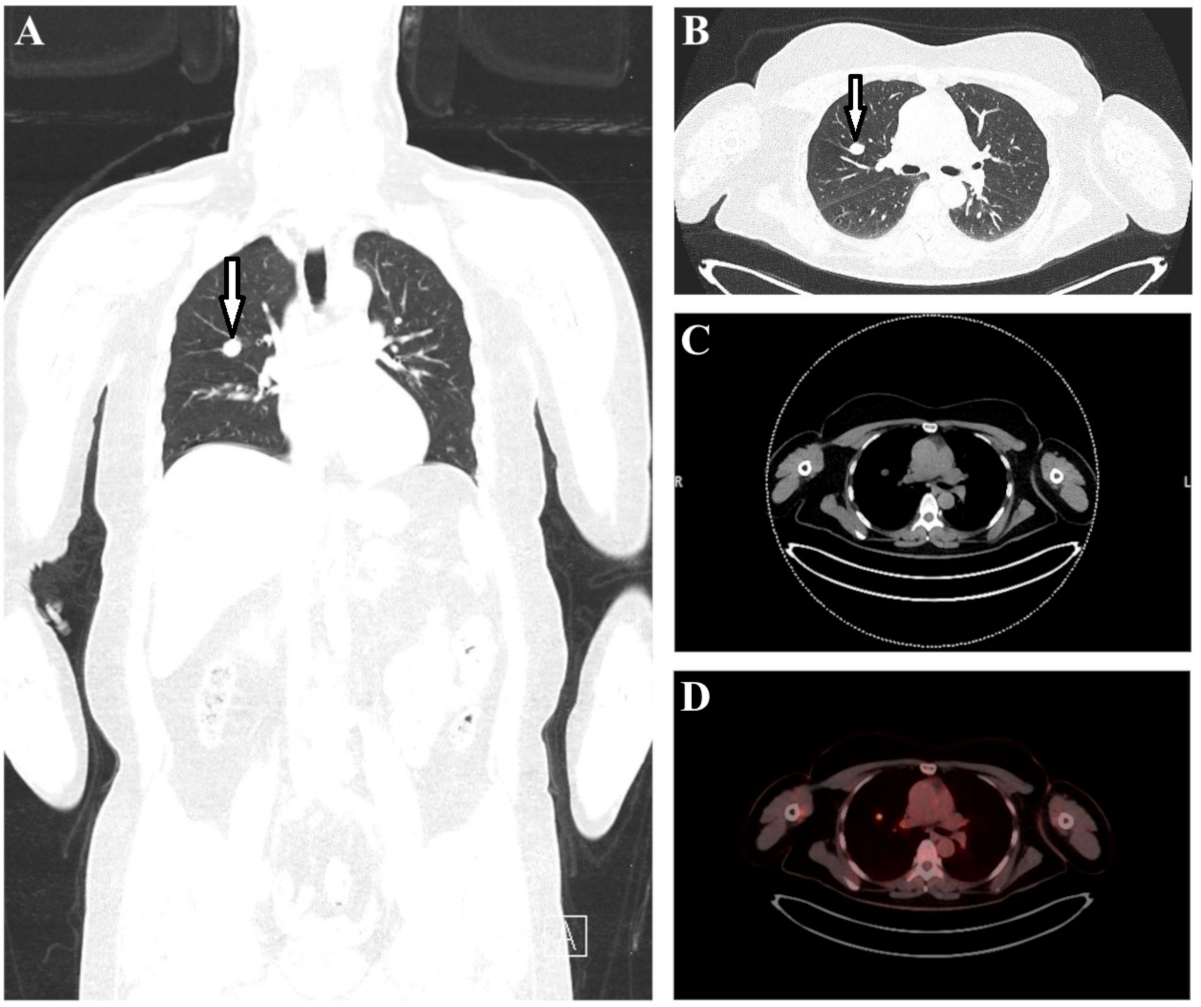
The multiplanar reformate image (A) and lung CT (B) revealed a 16 mm x 12 mm mass in the right upper lobe (marked with arrows). Axial CT (C) and transaxial fused PET-CT image (D) showed an increased focal ^18^F-FDG uptake (SUVmax 6.3) in the right upper lobe parahilar.
